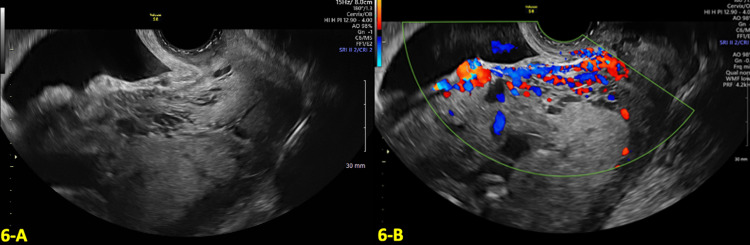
Transvaginal ultrasound (TVUS) findings at 23 weeks and one day gestational age (GA) A: Anterior placenta previa with placenta lacunae and an irregular bladder line highly suspicious for percreta at 23 weeks and one day gestational age (GA). B: Increased vascularity on Color Doppler transvaginal ultrasound (TVUS) at the uterine-serosa bladder interface at 23 weeks and one day GA.
